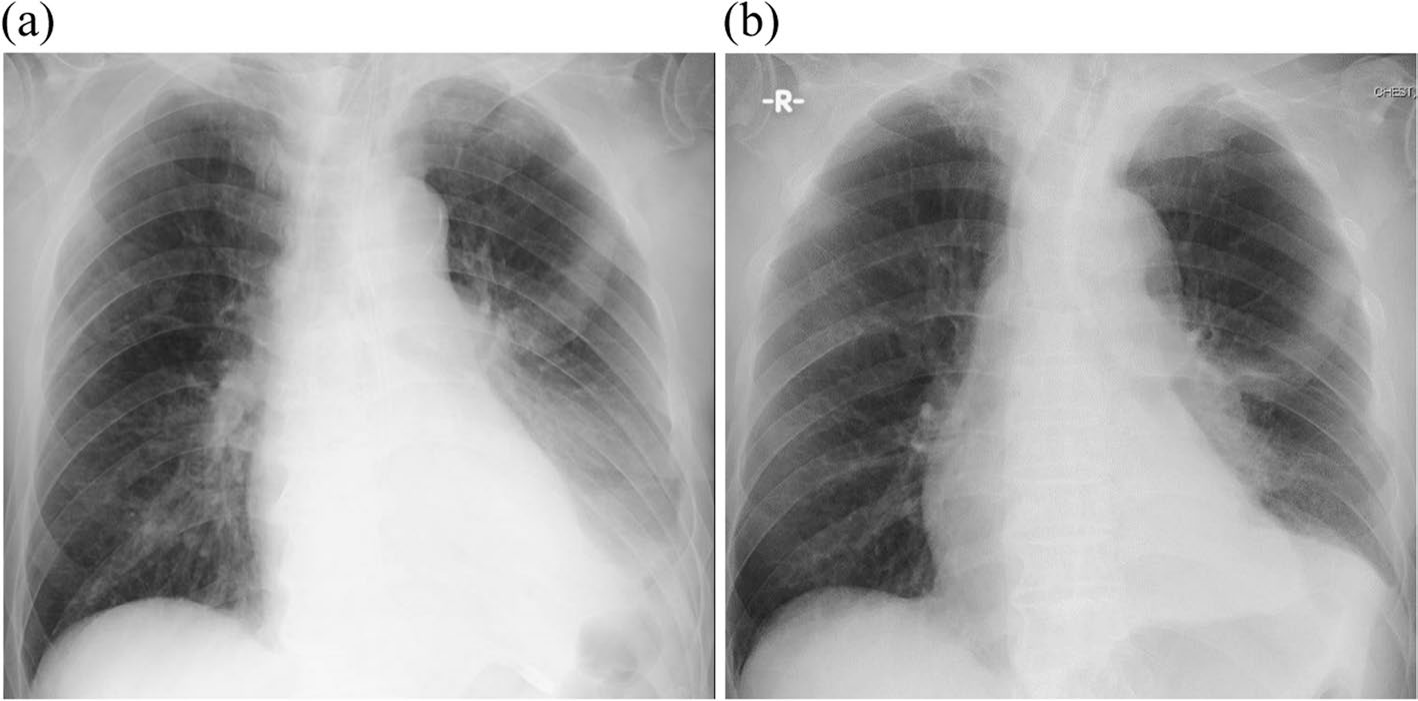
Chest radiograph showing reappearance of atelectasis in the left lower lung field on day 19, after extubation (a), and on day 30, after resolution following resumption of intrapulmonary percussive ventilation (b)
